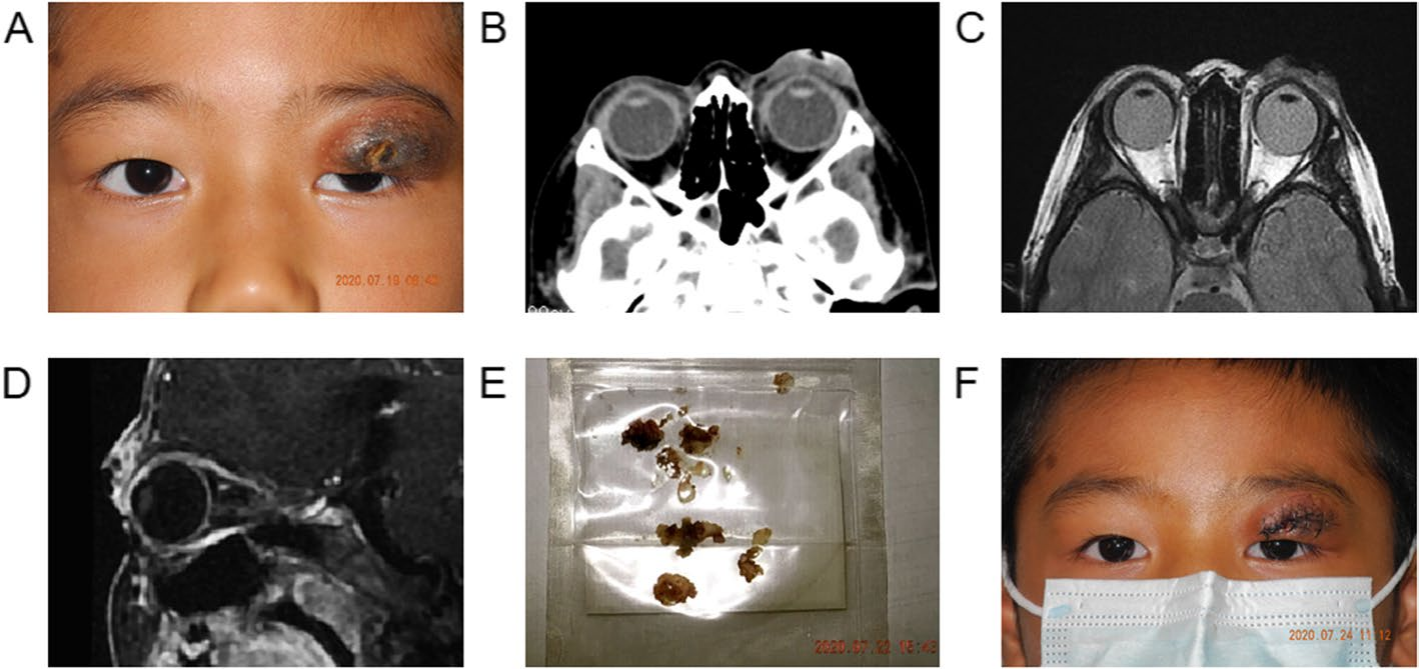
Case 2. A Appearance on presentation showing swelling of the left upper eyelid with a chronic discharging sinus, necrosis and slight ptosis. B CT scan of the orbits showing extensive swelling and increased density of the subcutaneous tissue of the left upper eyelid. C On T2 axial and sagittal plain MRI, the left upper eyelid was irregularly thickened, showing mixed and slightly hyperintensity, and the edge was unclear. Small strips of hypointensity were seen in the anterior part of the lesion, and the edge was unclear. D On sagittal enhancement of T1, uneven and obvious enhancement was seen in the left upper eyelid area, and no enhancement was seen in the anterior hypointensity area of the lesion, and the edge was clear. E Solidified grease and abnormal tissue surgically removed from the the orbicularis and orbital fat through the palpebral incision. F Appearance on the seventh day after surgery showing reduced swelling and ptosis of the left upper eyelid
